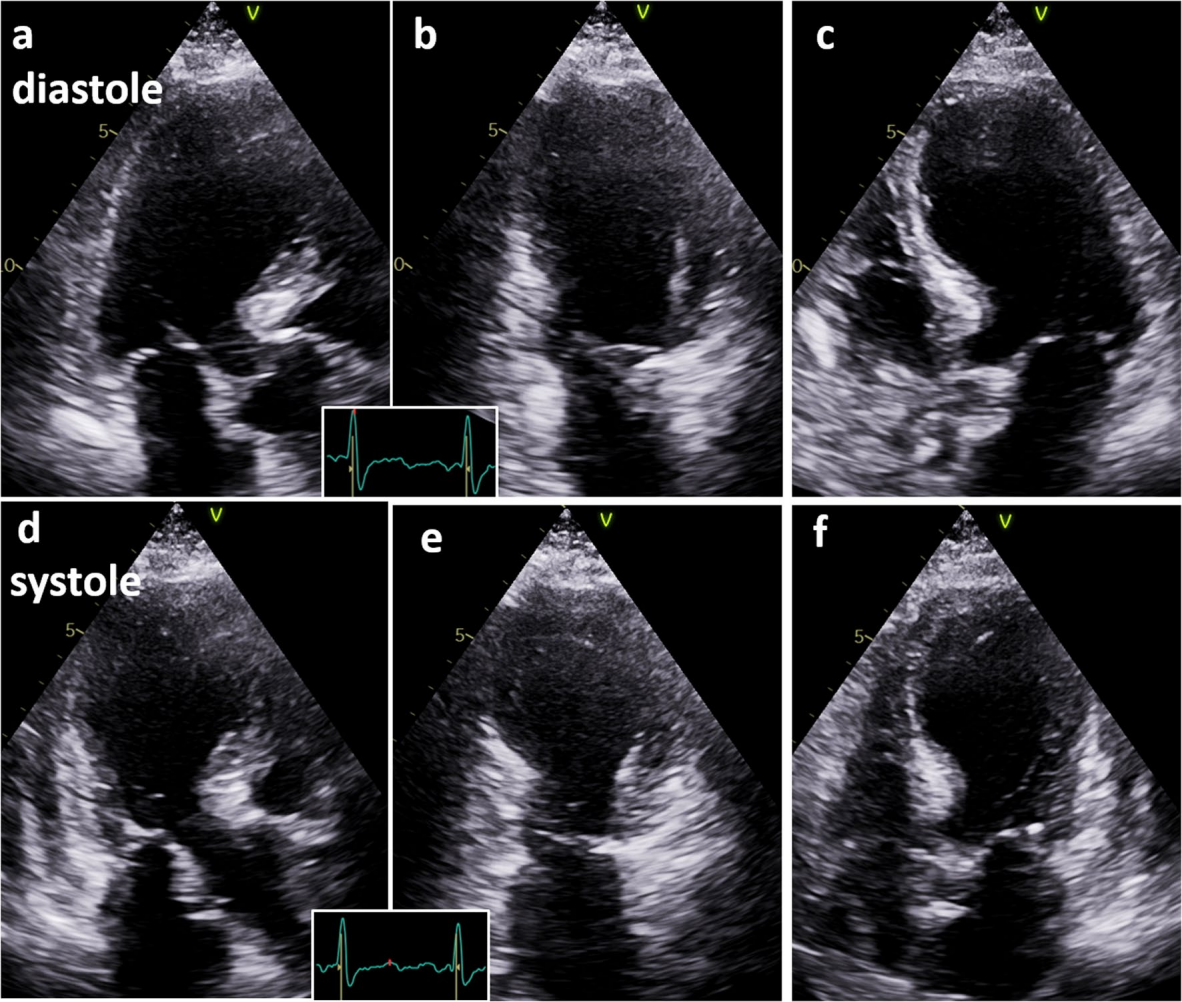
Echocardiography showed wall motion abnormalities with typical apical ballooning documented by apical long axis view, 2- and 4- chamber view during diastole (a-c) and systole (d-f)
